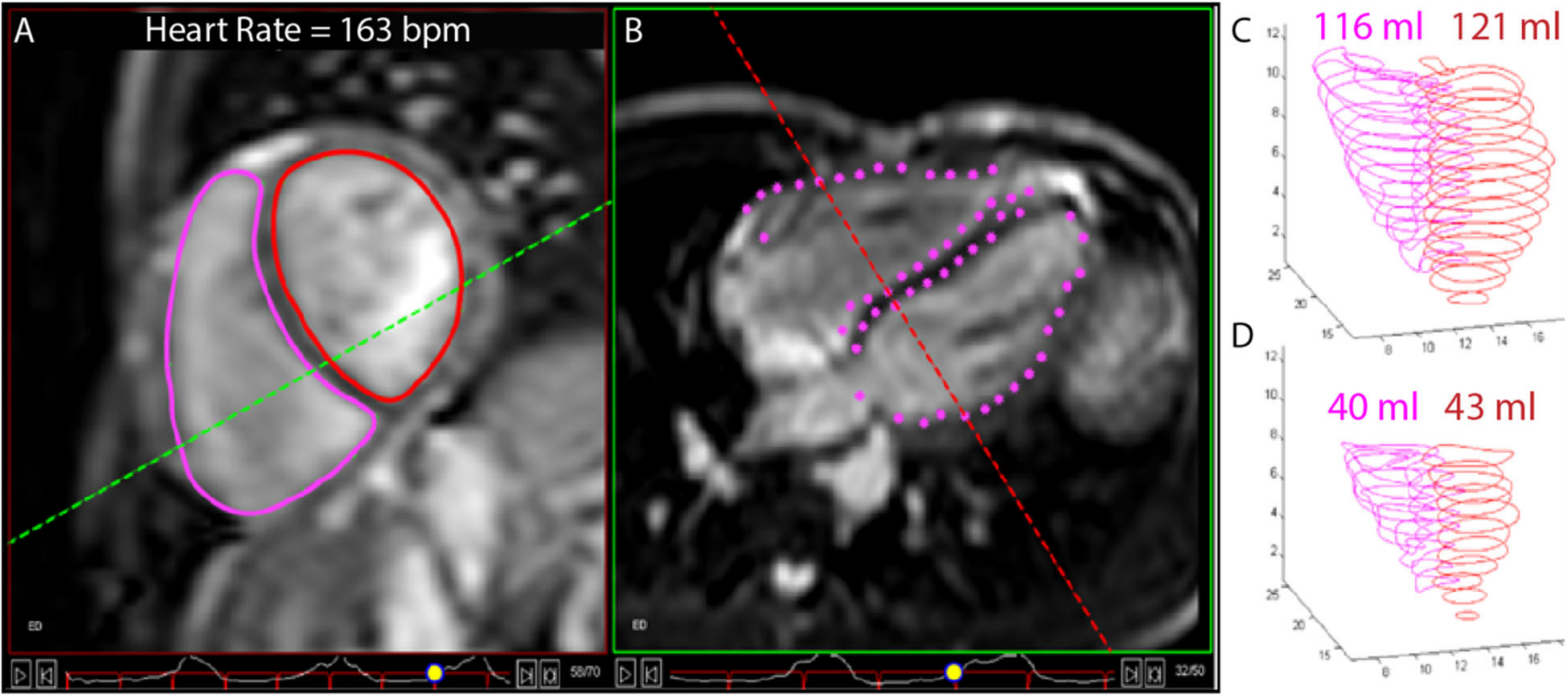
Example of real-time ungated exercise cardiac MRI imaging during high-intensity exercise. a Short axis images are used to define the endocardial borders for the calculation of ventricular volumes. The point at which these transect the horizontal long-axis plane (b) is shown by the pink dots at the line of the red dotted line. This allows for cross-checking for the accuracy of endocardial contours and for the determination of the atrio-ventricular level on the short axis images. The endocardial ventricular borders for each short-axis slice at (c) end-diastole and (d) end-systole are summed to determine end-diastolic and end-systolic ventricular volumes respectively. This process is performed for images taken at rest and all intensities of exercise
